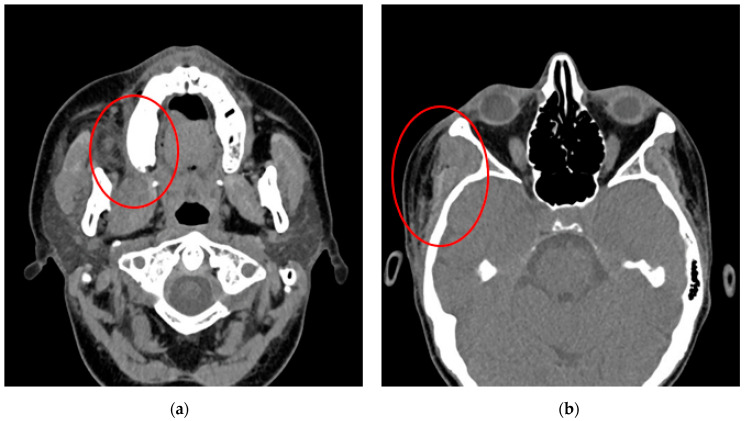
CT scans of a 34-year-old pregnant woman showing facial phlegmon involving the right pterygomandibular (a) and temporal spaces (b) (red circles).
